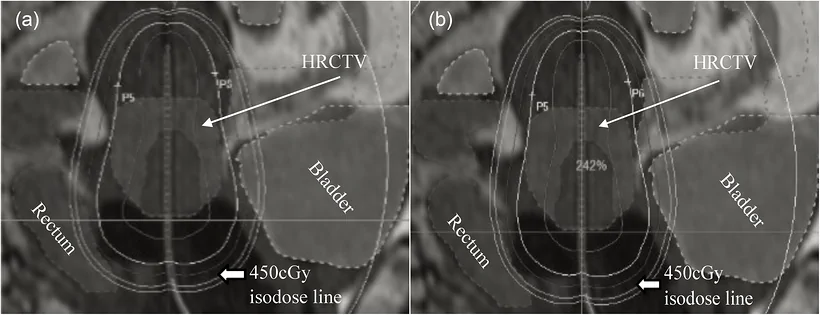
A sagittal MRI view displays isodose lines overlaid on the anatomical structures in intracavitary brachytherapy. (a) The initial treatment plan (b) The re‐optimized treatment plan.
